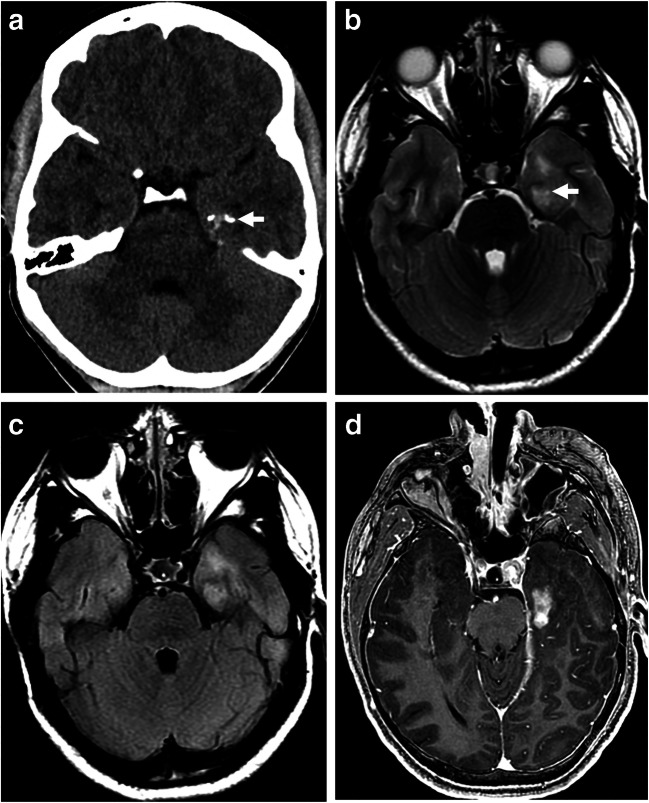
Ganglioglioma. a Axial non-contrast CT scan through the hippocampus, demonstrating a few punctate calcifications in the left hippocampus (arrow). No obvious tumor is identified. b An axial T2-weighted image through the same level, demonstrating ill-defined T2-hyperintense tumor (arrow) in the left hippocampus. The T2 abnormality extends to the left anterior temporal region. c An axial T2 FLAIR image through the same level better demonstrates the T2 abnormality in the left medial and anterior temporal lobe. Of note, there is no appreciable mass effect for the size of the tumor. d An axial postcontrast 3D T1-weighted image through the same level better shows heterogeneous enhancement of the hippocampal component of the tumor. The more anterior component does not show any abnormal enhancement
